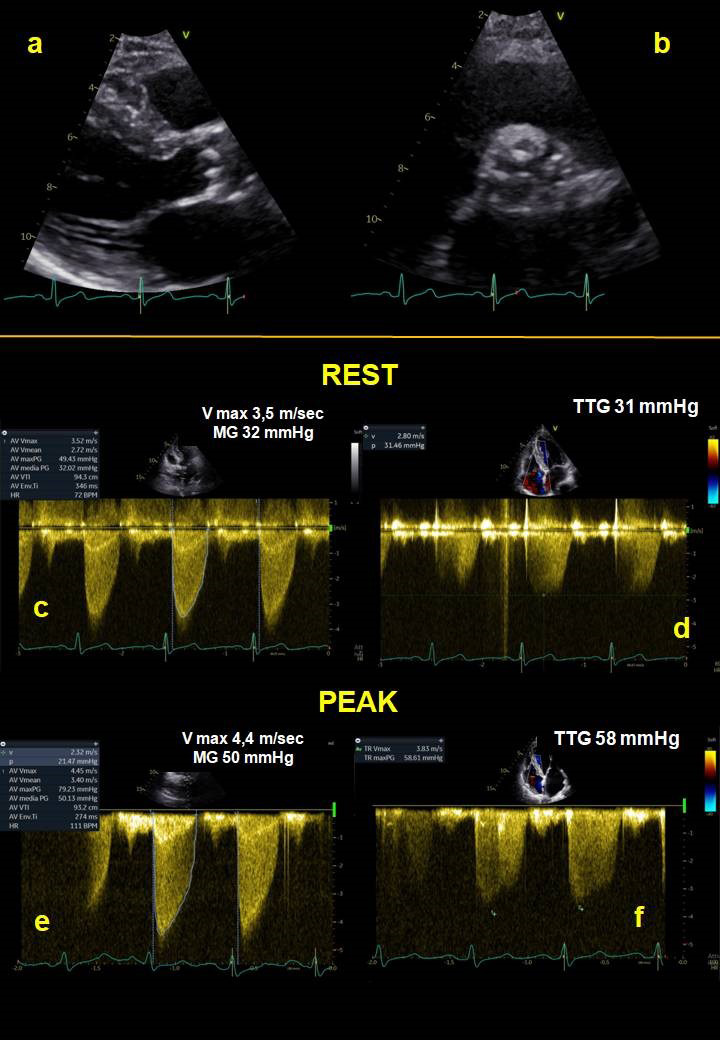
Aortic stenosis. Stress-echocardiography in a patient affected
